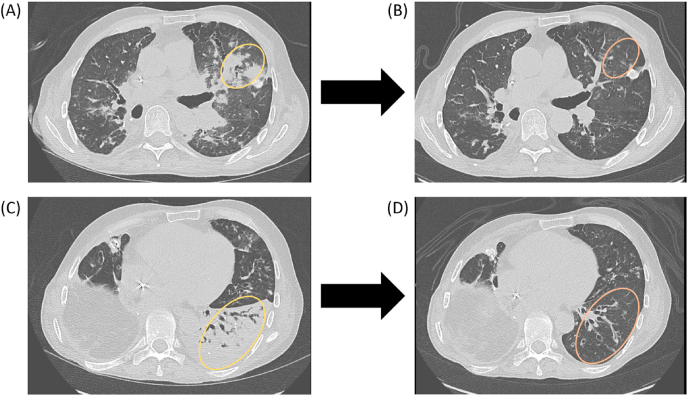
Autoimmune pneumonitis and its improvement following lymphocyte-directed immunomodulation. A-B. Chest CT showing left upper lobe consolidation (A, yellow oval) with interval improvement following immunomodulatory treatment (B, orange oval). C-D. Chest CT showing left lower lobe consolidation with bronchiectatic air bronchograms (C, yellow oval) with interval improvement (D, orange oval) following immunomodulatory treatment. (For interpretation of the references to colour in this figure legend, the reader is referred to the Web version of this article.)
